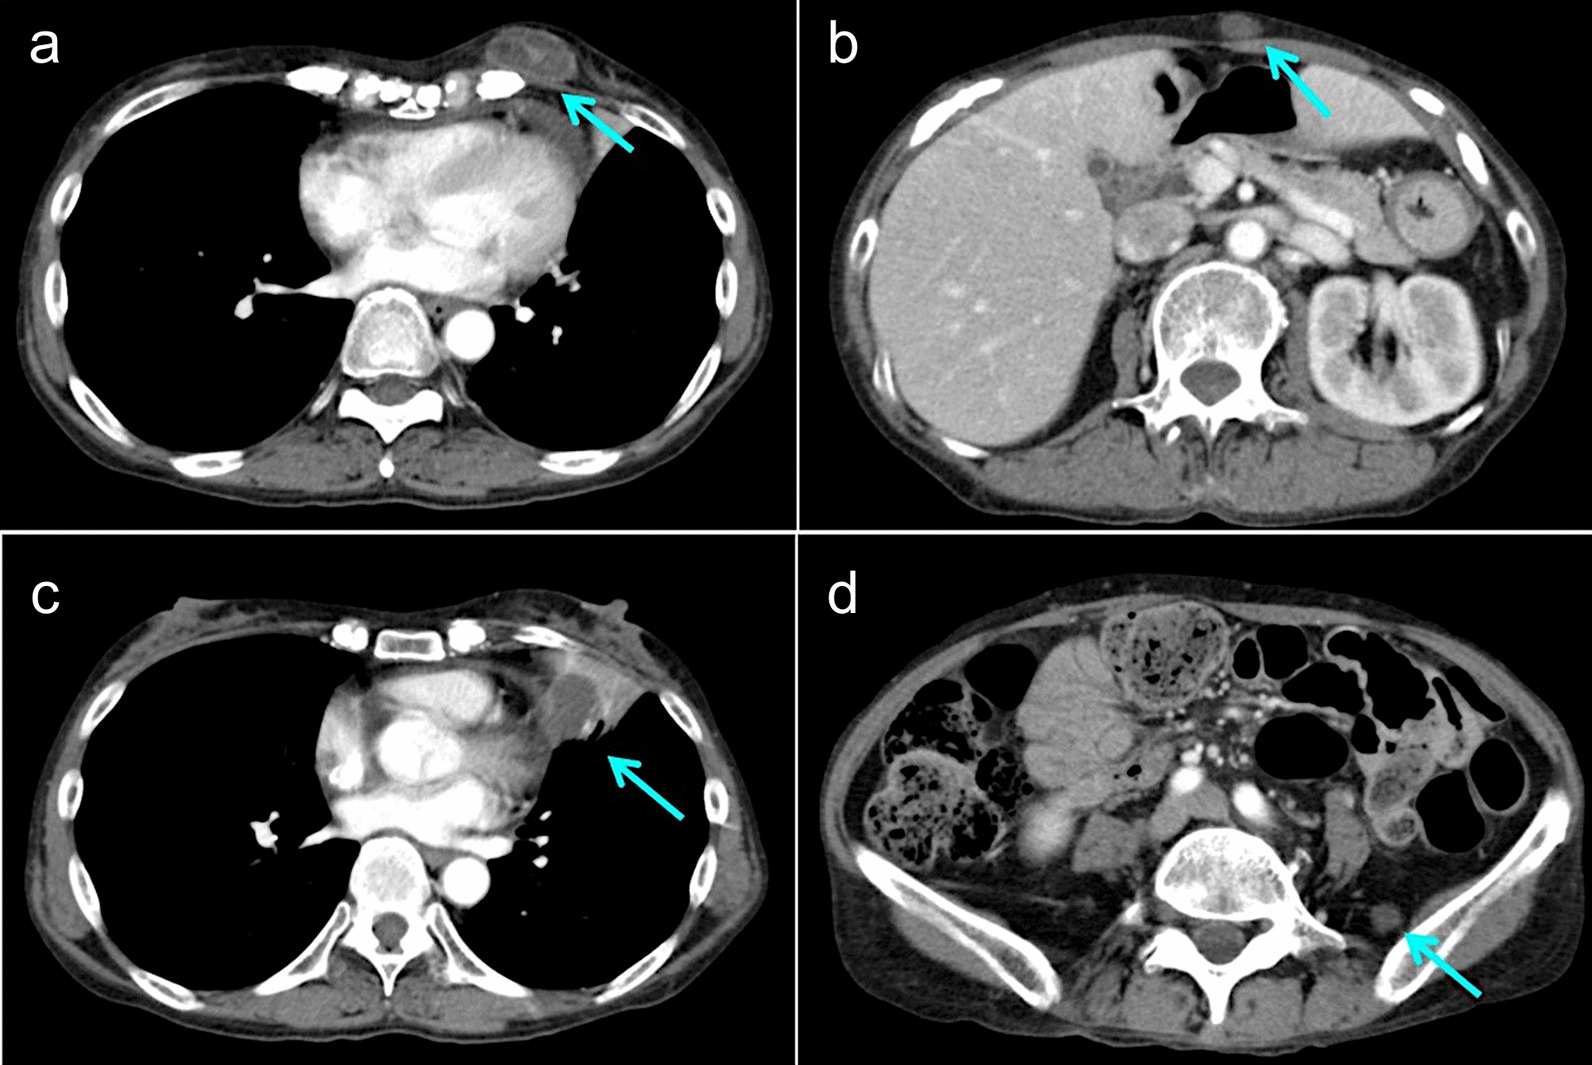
Contrast-enhanced computed tomography scan imaging a a mass in the breast (arrow), b a subcutaneous nodule in the abdomen (arrow), c a mass in the left lung (arrow), d a nodule in the left retroperitoneum (arrow)
